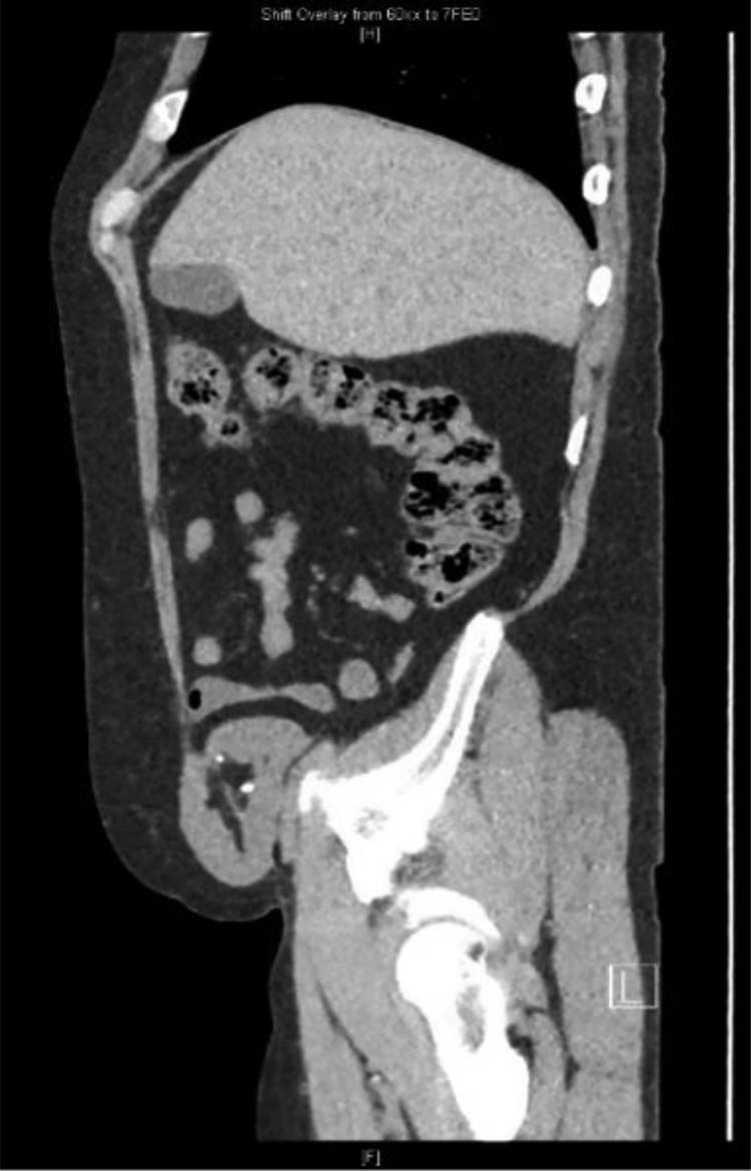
Sagittal view CT: herniation of the transplanted kidney through the right abdominal wall.
